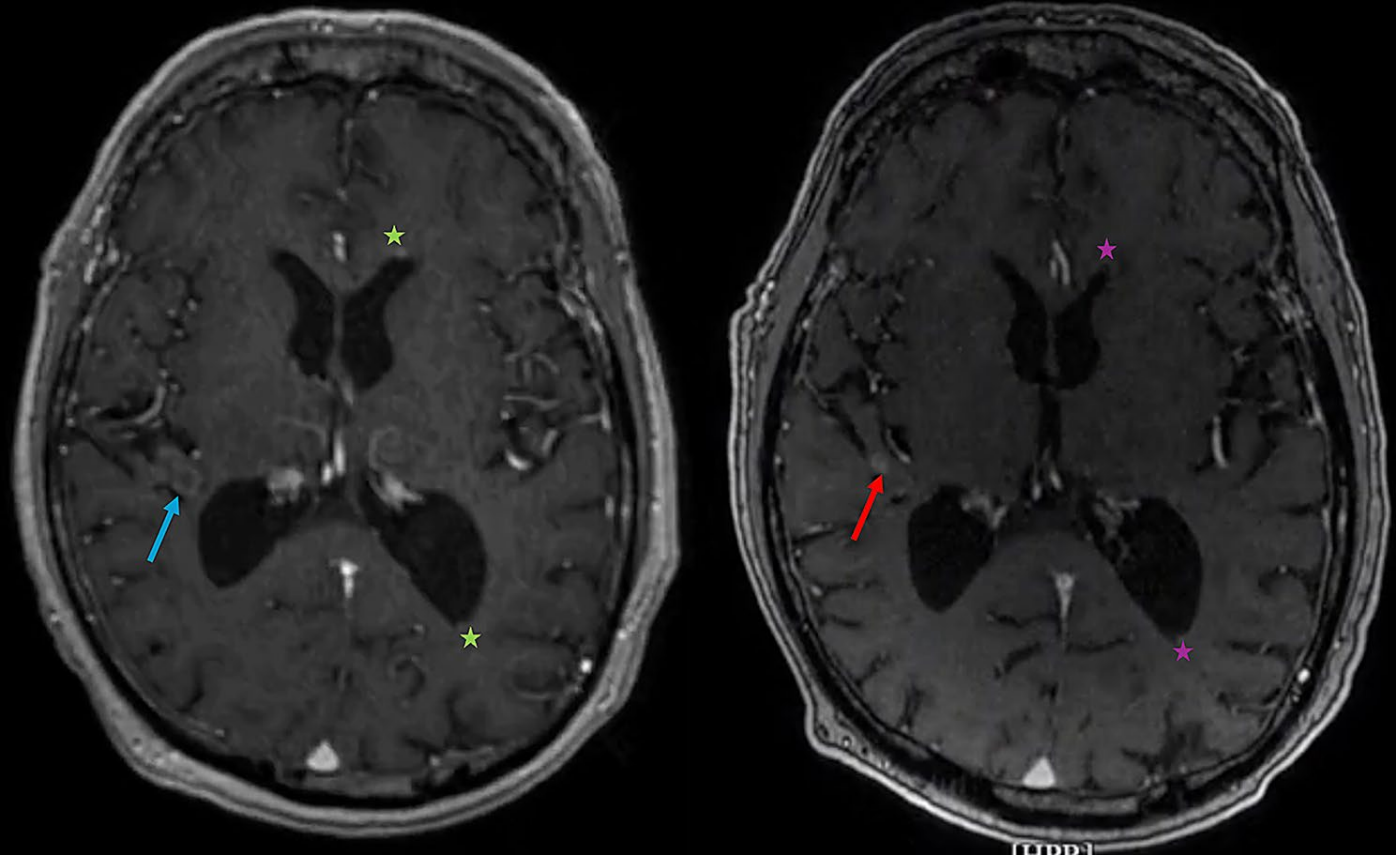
Postoperative and follow-up MRI imaging. Comparison of the postoperative T1-weighed post-contrast axial MRI sequence prior to discharge of the patient (left) with the one-year-follow-up T1-weighed post-contrast axial MRI sequence. The residual perilesional contrast enhancement on the postoperative imaging (blue arrow) shows signs of regression and consolidation on one-year-follow-up (red arrow). Patches of contrast enhancement in the anterior and posterior horns of lateral ventricles that were seen on the postoperative imaging (green asterix) are also consistent with regressing ventriculitis on the one-year-follow-up MRI (violet asterix). MRI = Magnetic Resonance Imaging
